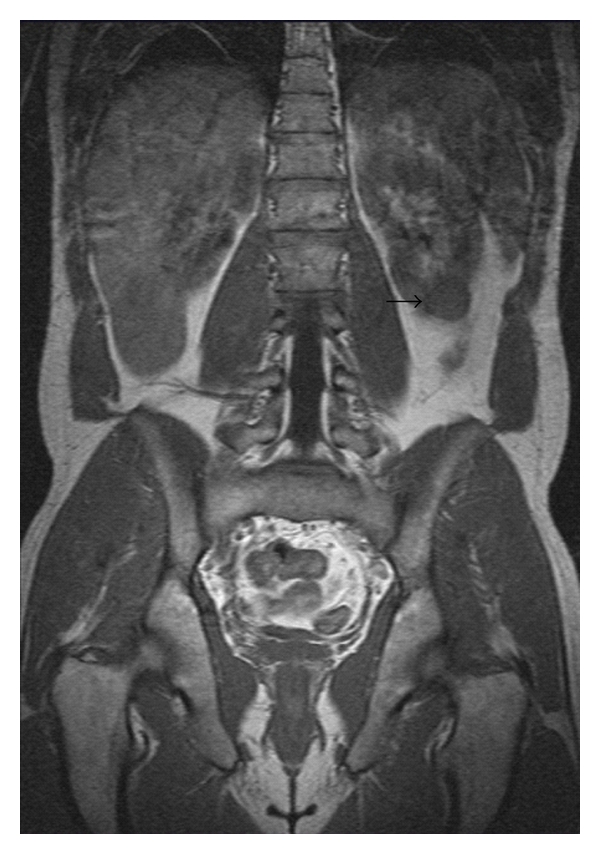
Coronal magnetic resonance imaging scan demonstrating 30 mm left renal mass (arrow).
